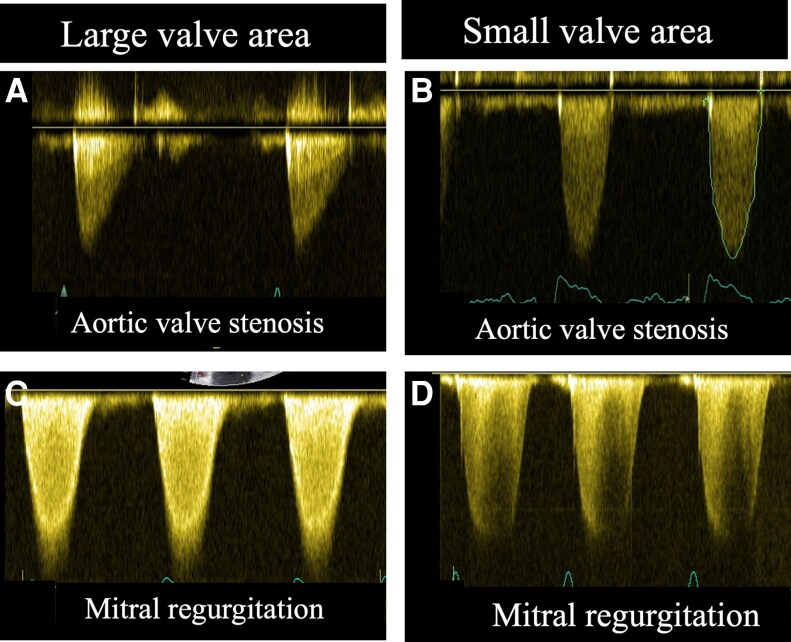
Impact of valve area on the continuous-wave Doppler shape. Impact of the stenotic or regurgitant orifice area on the CWD signal shape in AS (*A* and *B*) and MR (*C* and *D*). In general, a larger area generates an early peaking and triangular signal (*A* and *C*), whereas a relative smaller area generates a more parabolic, midsystolic peaking Doppler signal (*B* and *D*).
